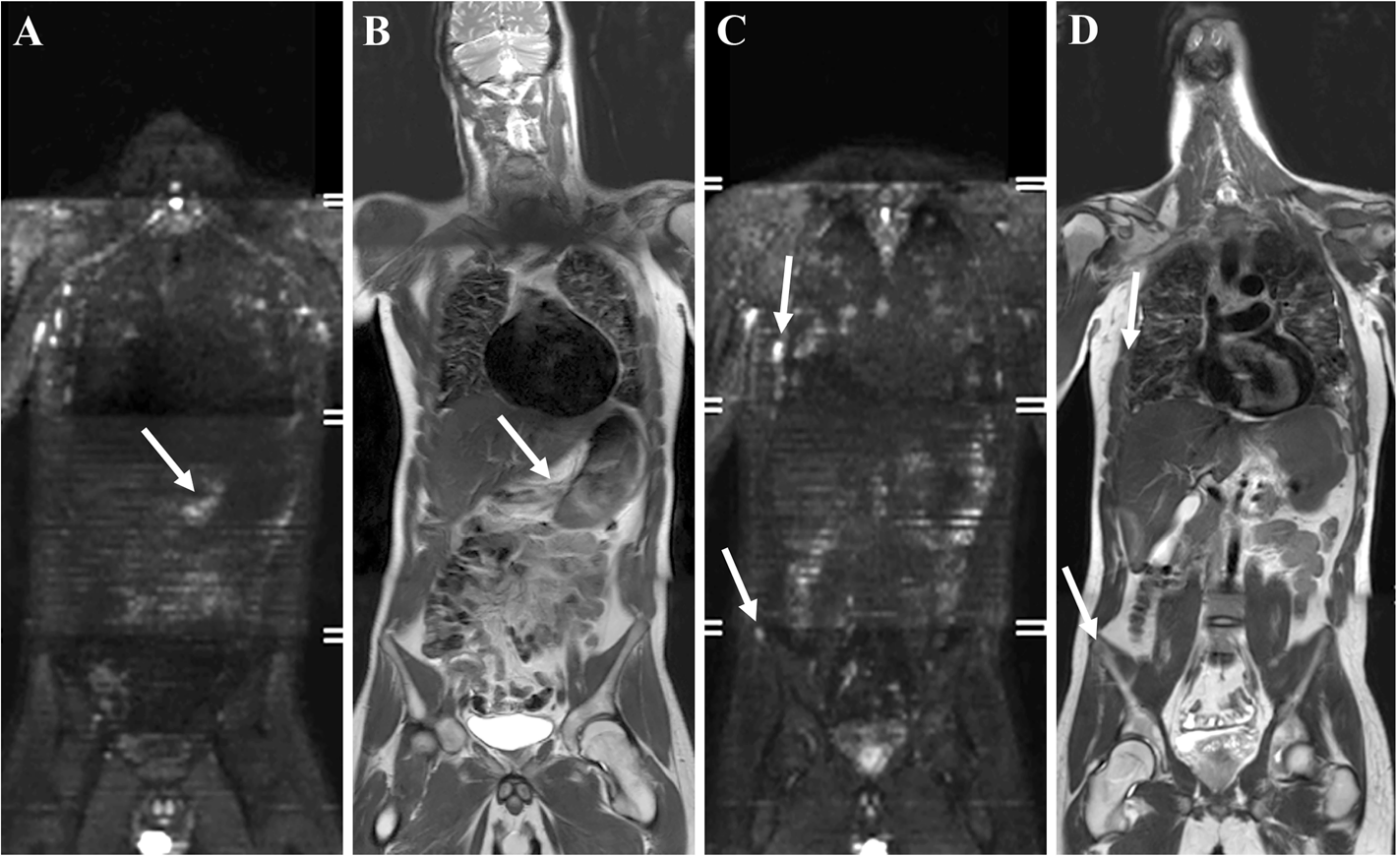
(A,B) WB-DWI with b1000-images and T2-weighted images in the coronal plane shows a tumoral mass in the lesser curvature of the stomach (arrow), (C,D) with diffuse metastases in the lungs and mediastinum as well as skeletal metastases in a right-sided rib and right iliac wing (arrows).
